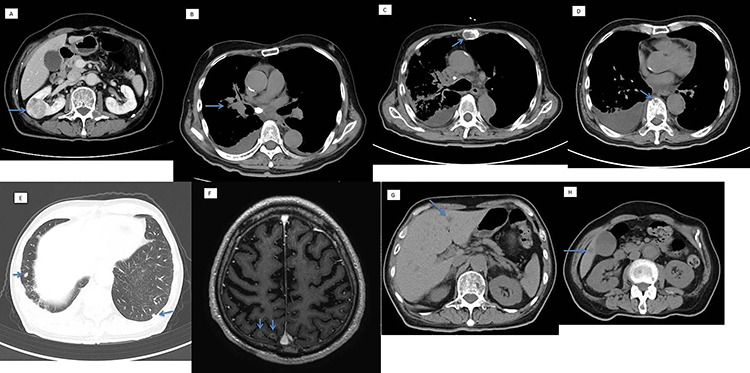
Computed tomography scan showing (A) a 38-mm right renal mass (suspected renal cancer), (B) right hilar lung cancer, with metastasis in the (C and D) bone, (E) lungs, (F) brain and (G) liver.
